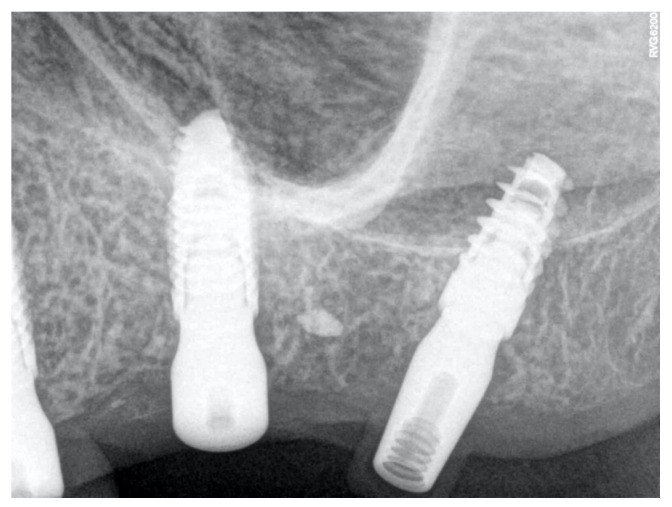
Radiography of the implant after 45 days of healing.
